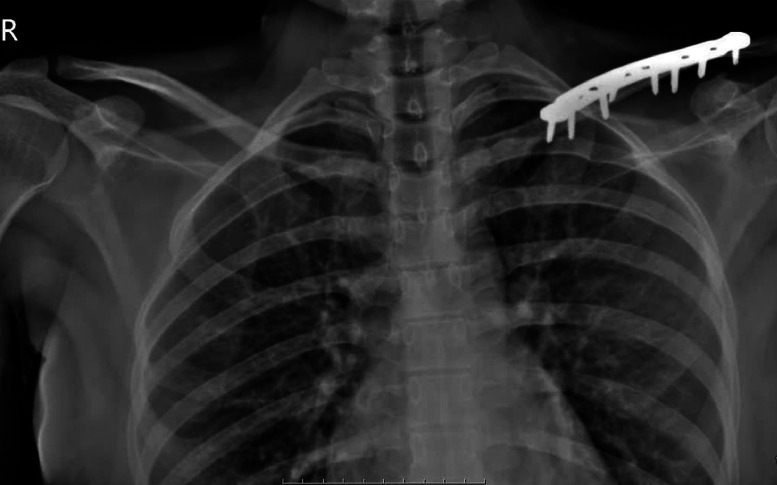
Postoperative X-ray of the clavicle after ORIF with plates and screws.
